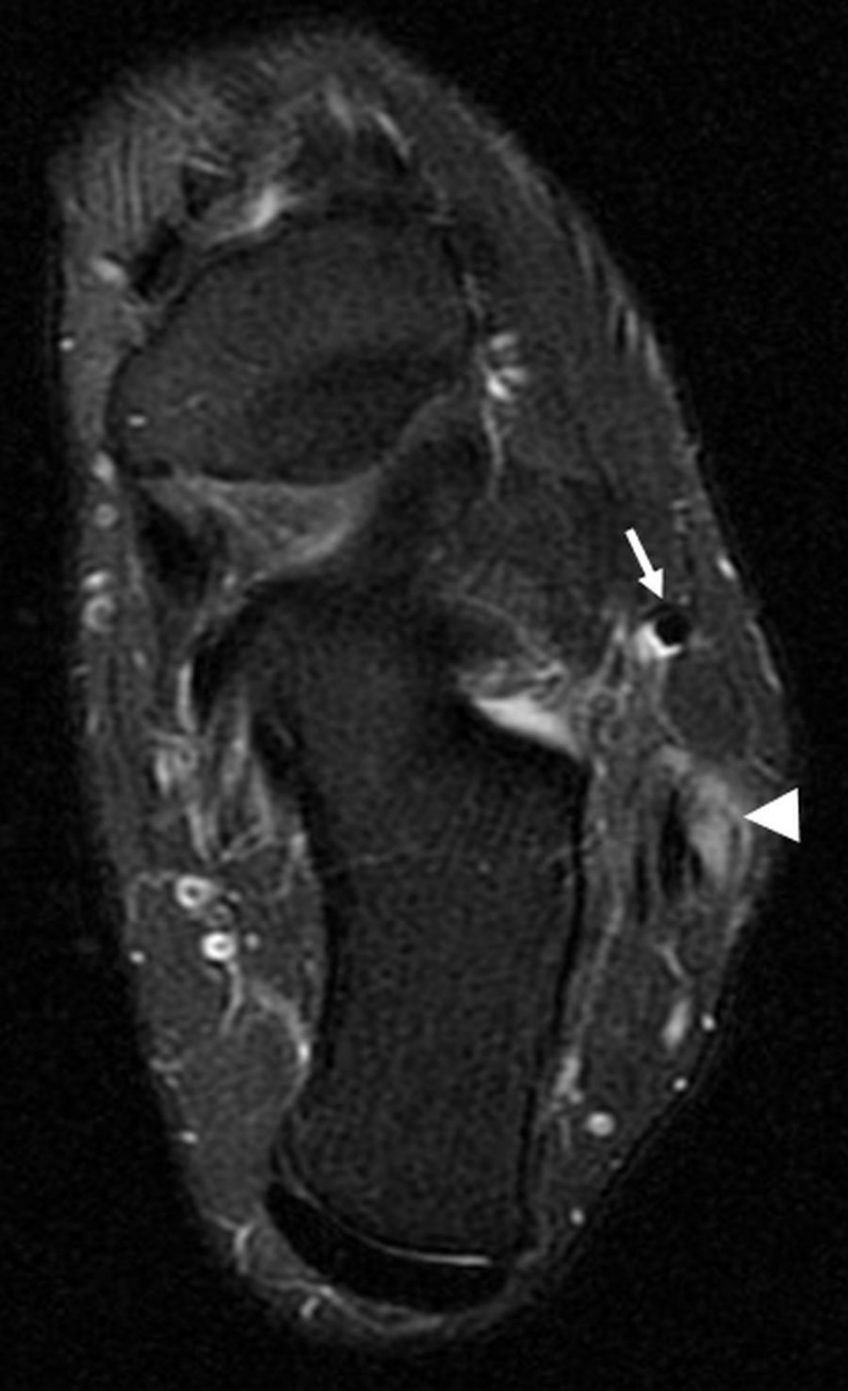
Tenosynovitis of the Common Peroneal Tendon Sheath and Peroneus Tertius Axial T2-weighted fat suppressed image inferior to the lateral malleolus shows T2-hyperintensity surrounding both the common peroneal tendon sheath (arrowhead) and the peroneus tertius (arrow), consistent with tenosynovitis.
